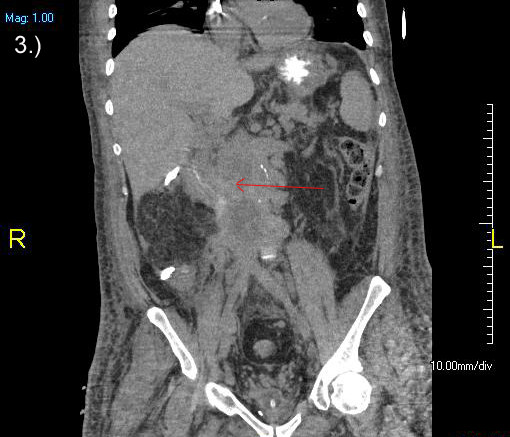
Computed tomography scan demonstrating extensive metastatic disease of the retroperitoneum.
